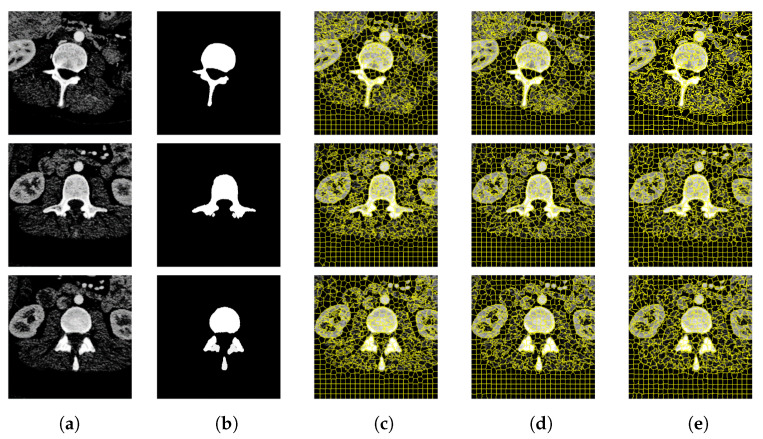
CT image segmentation of vertebrae. (a) Original image. (b) True image. (c) SLIC. (d) SNIC. (e) Proposed.
